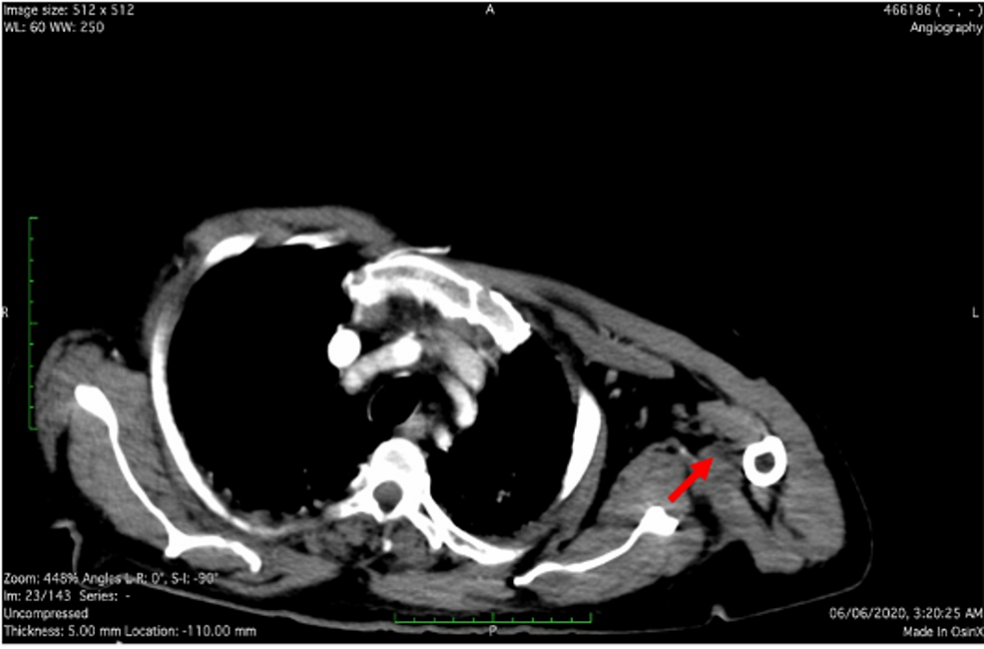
Axial section of CT aortogram showing filling defect within the left axillary artery (red arrow).
